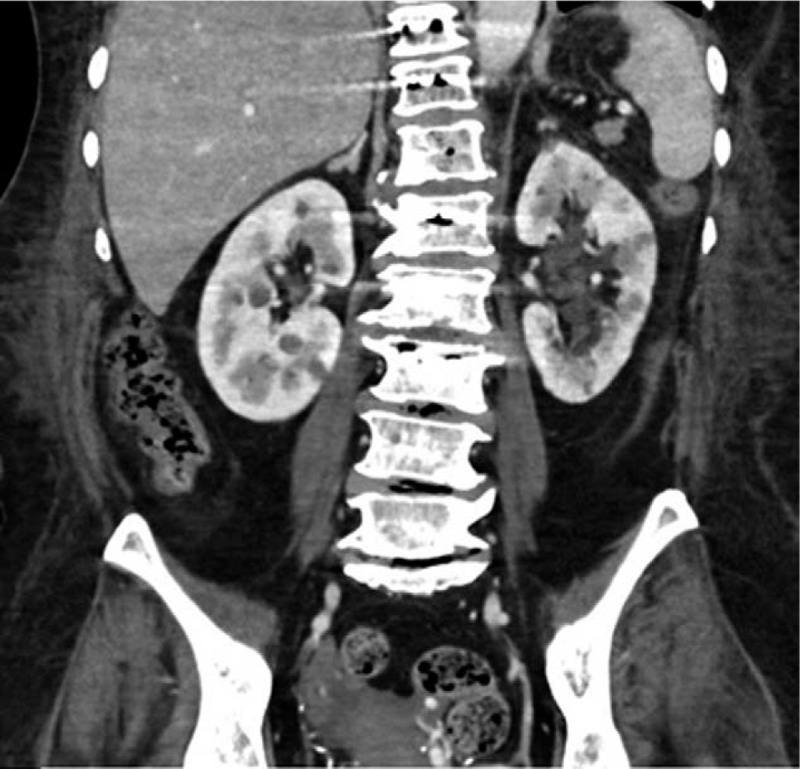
Last follow-up computed tomography scan in coronal plane showing improved right psoas abscess.
